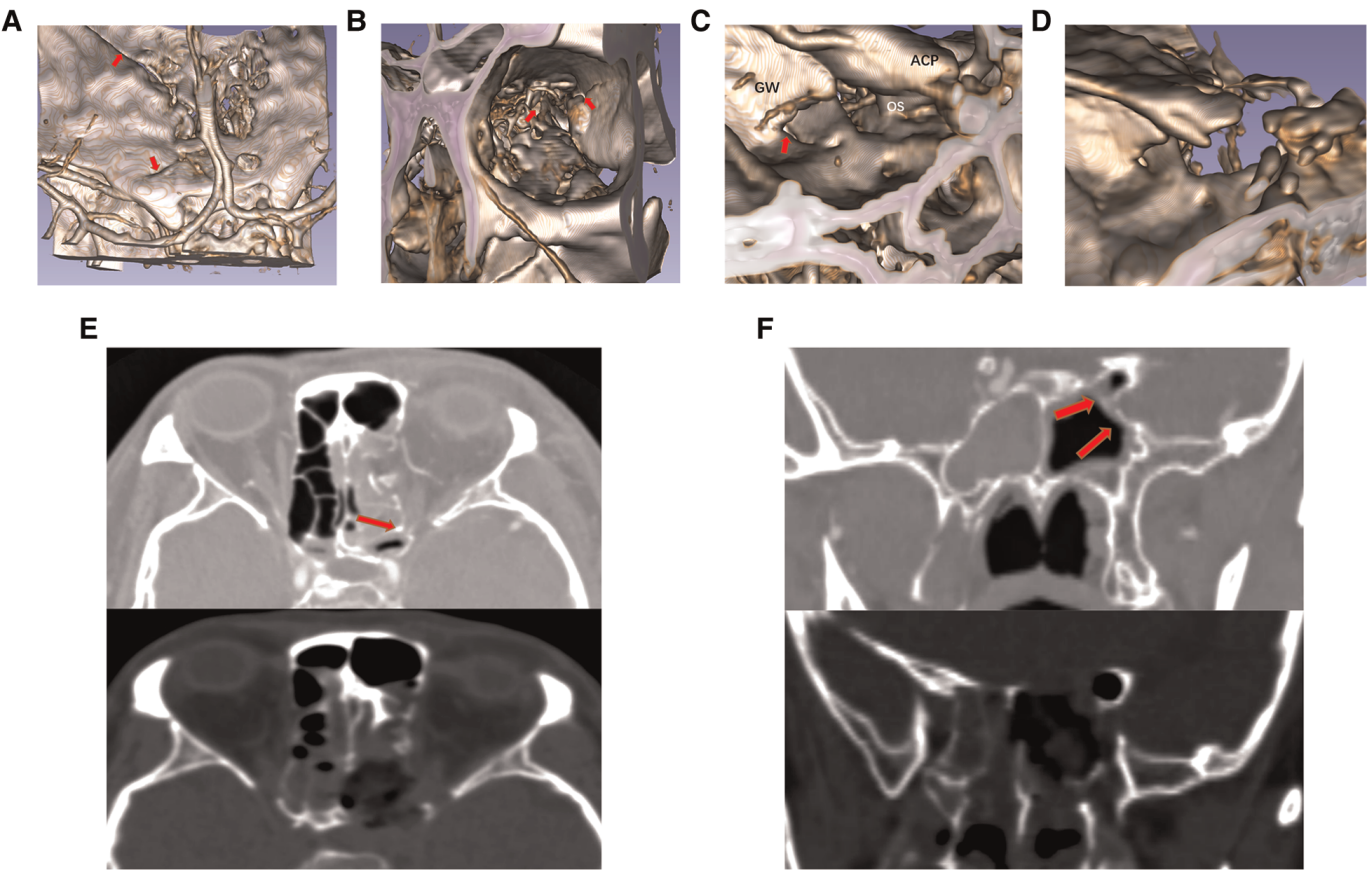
Comparison of preoperative and postoperative imaging data. 3D views of patients’ left orbit from different angles are shown in (A–C). The red arrow indicates the fracture line. (A) The fractures of the small wing of the sphenoid bone involve the superior wall of the optic nerve. (B) Fracture line of the superior orbital fissure from the orbital perspective. (C) Observation of the fracture line of the superior orbital fissure from the upper left of the skull base. (D) Thin-slice CT 3D reconstruction image after surgery. The preoperative (top) and postoperative (bottom) images of the patients are shown in E-F. The red arrow indicates the fracture line. (E) Comparison of preoperative and postoperative cranial axial CT findings. (F) Comparison of preoperative and postoperative coronal cranial CT findings. The upper red arrow indicates the optic canal fracture line, and the lower red arrow indicates the fracture line on the superior medial wall of the superior orbital fissure (corresponding to the oculomotor nerve fissure segment).
